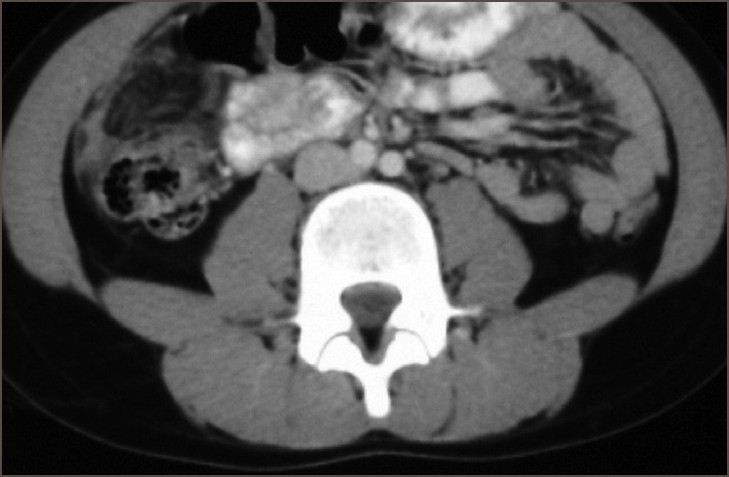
CT scan showing fat stranding anterior to Cecum and ascending colon in RIF
